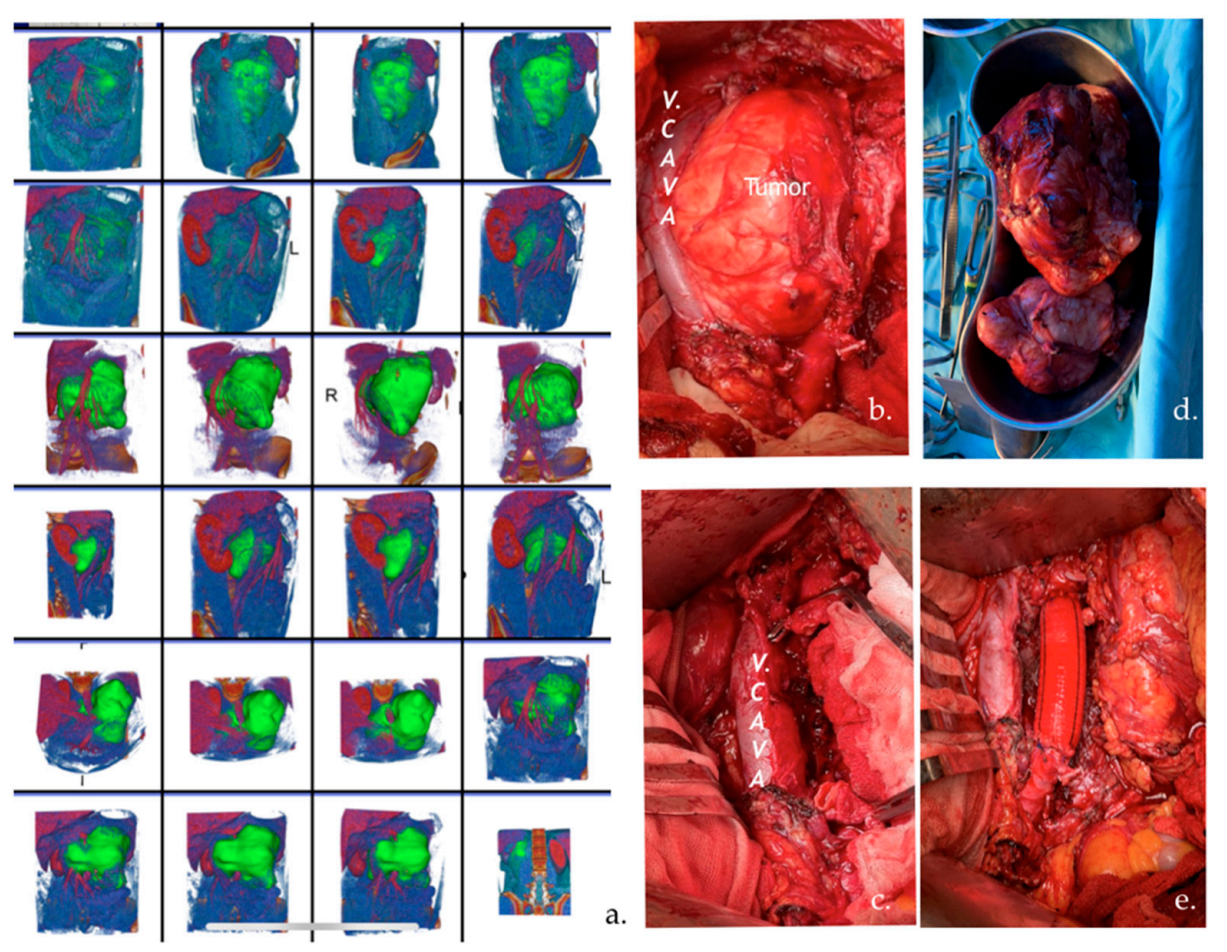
(a) CT images with 3D segmentation of a 30-year-old patient with an enlarged left pericaval lymph node (LN) after chemotherapy. The patient underwent PC-RPLND. The volume of LN was 15 cm in diameter. (b,c) Pictures of intraoperative retroperitoneal surgery of the tumor that displaced the v. cava and infiltrated the aorta. (d) The tumor was totally resected. (e) Aortic vascular prosthesis.
